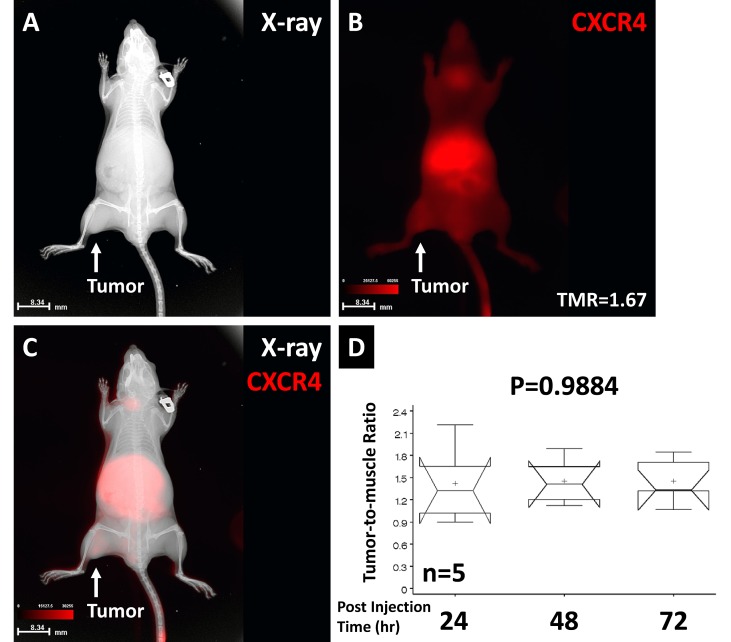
The CXCR4 agent binding to the NPC tumor X-ray imaging showed the tumor location (A). The CXCR4 signal showed whole-body distribution and a high TMR in the tumor region (B). Overlaid anatomic and optical images showed the location of the CXCR4 signals (C). Comparison of TMRs over the 72-hour post injection period showed no significant difference. The high variation at the early time point suggested that the imaging agent required time to be detected in the tumor region (D).
